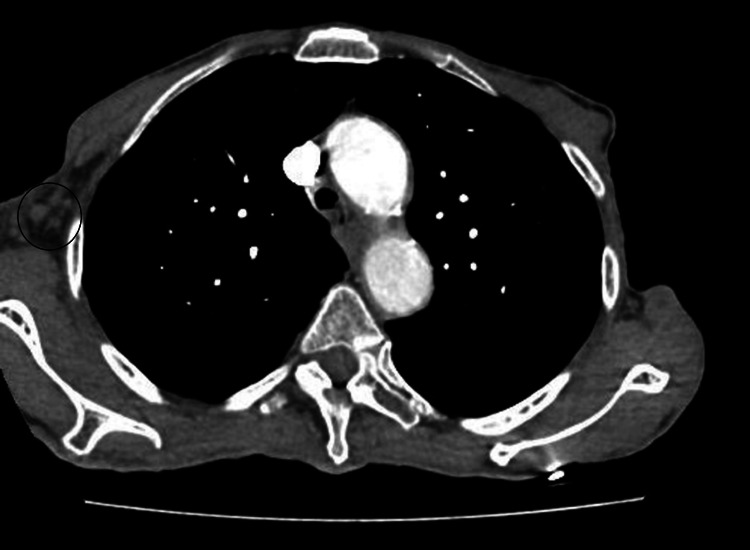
CT chest soft tissue window showing resolving enlarged right axillary lymph nodes (marked with a black circle) during chemotherapy on 11/27/2017
